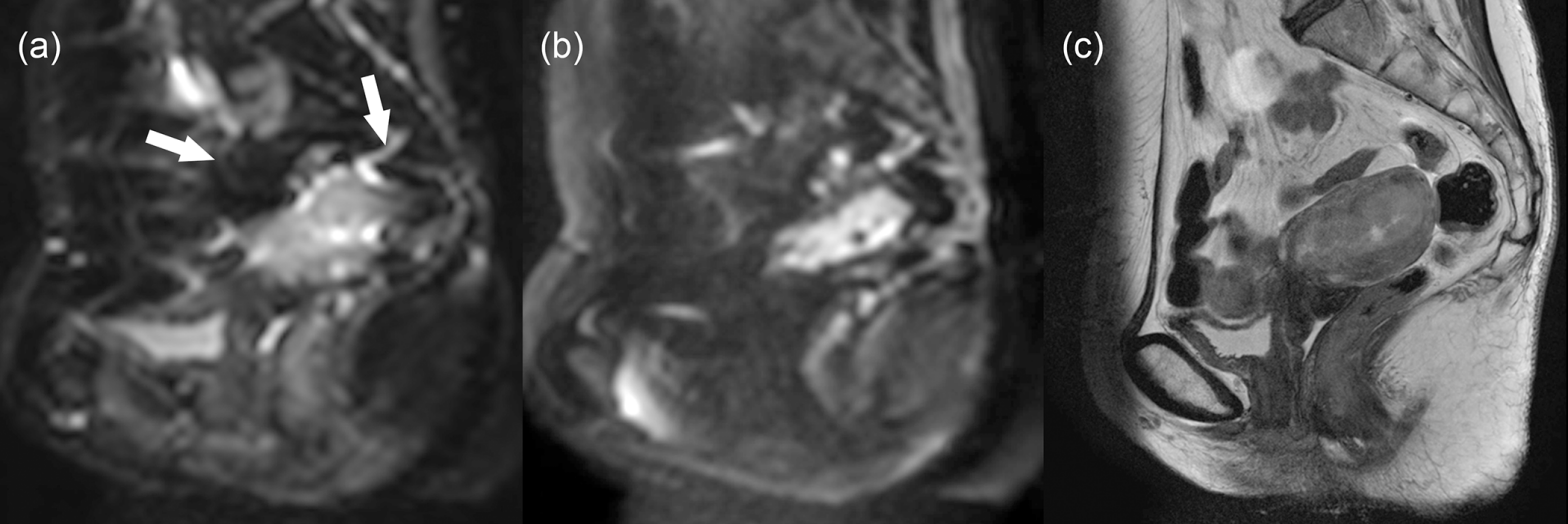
A 65-year-old woman with mixed carcinoma of the uterine corpus (serous adenocarcinoma, grade 2; endometrioid adenocarcinoma, grade 2). (a) Sagittal diffusion-weighted image at *b* = 0 s/mm^2^, (b) sagittal diffusion-weighted image at *b* = 2500 s/mm^2^, and (c) sagittal T2-weighted image show the tumor in the endometrium. Because of severe susceptibility artifacts due to bowel gas in the rectum and the sigmoid colon (arrows), it was hard to calculate diffusivity and kurtosis values for the myometrium. However, those values could be calculated for the endometrial tumor because of the lesser degree of artifacts at the center of the uterine corpus.
